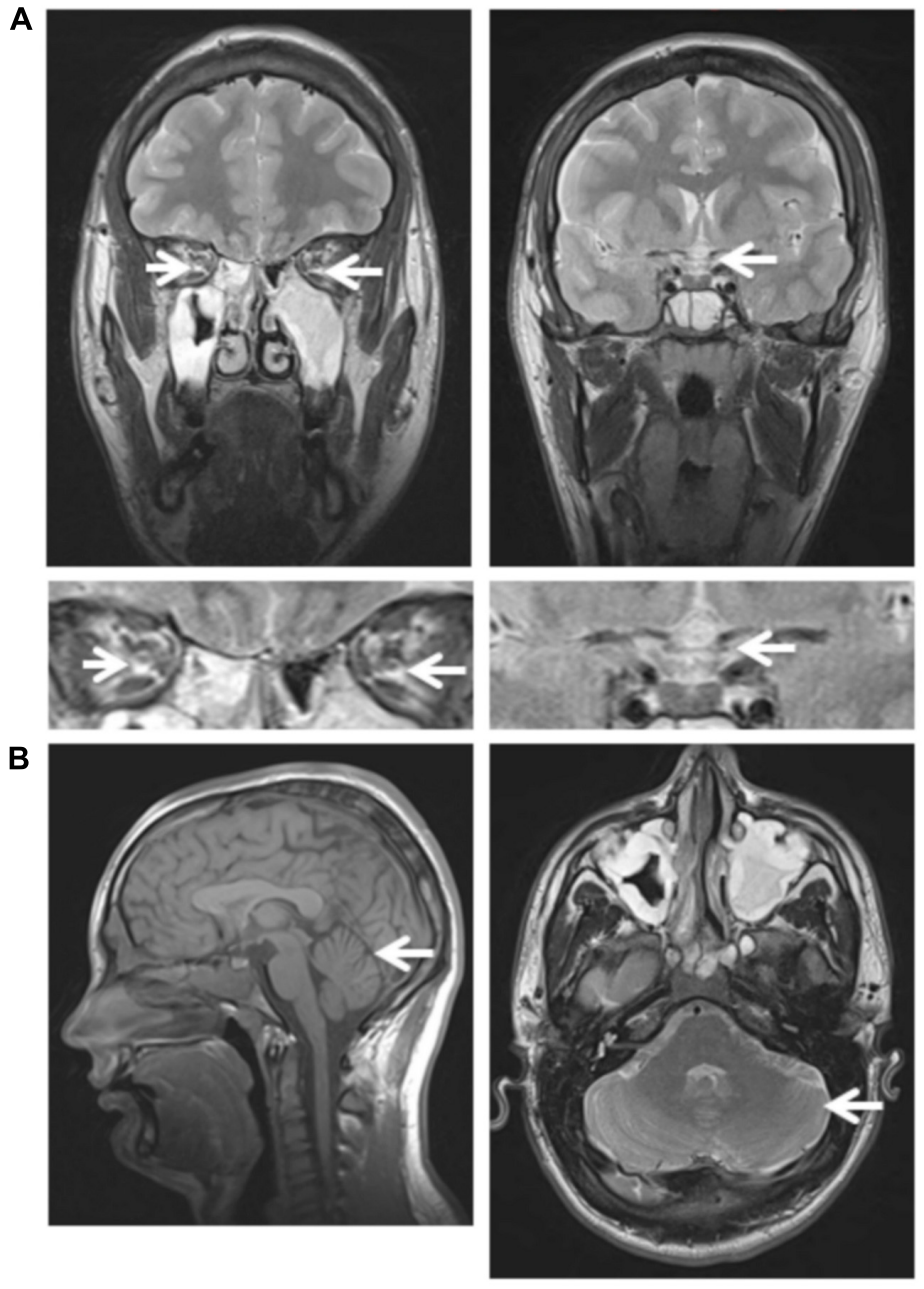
Brain MRI of patient III.4 at 18 years of age. (A) Left: Frontal section, T2 sequence, showing atrophy of the optic nerve (arrow) and Right: reduced size of the chiasma (arrow). Magnification of the optic track (Left) and chiasma (Right) regions are shown underneath the full picture. (B) Left: Sagittal section, T1 sequence, showing a normal aspect of the brain, especially of the vermis (arrow); Right: axial section, T2 sequence, showing a normal aspect of the posterior fossa, especially the cerebellar hemisphere (arrow).
